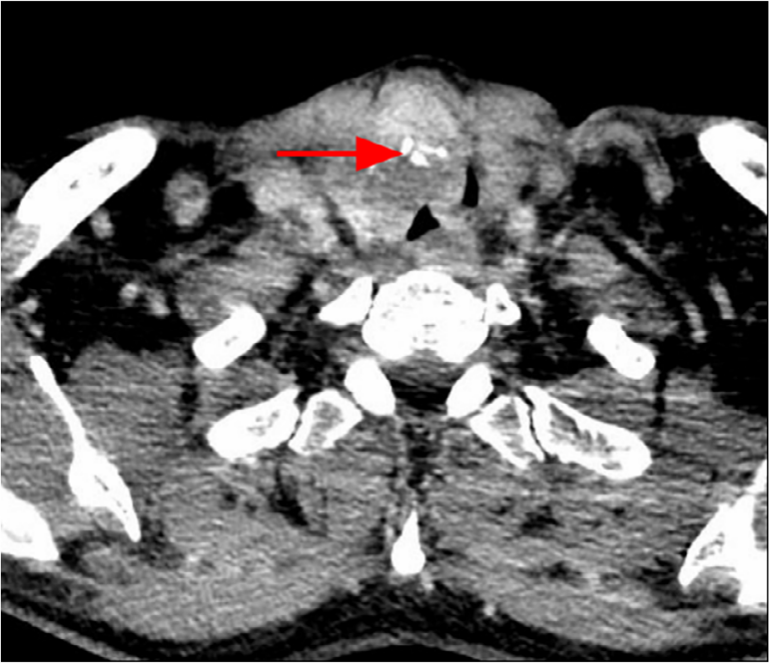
CT showing the extent and size of a tumor. The red arrow indicates thyroid carcinoma invading the trachea
